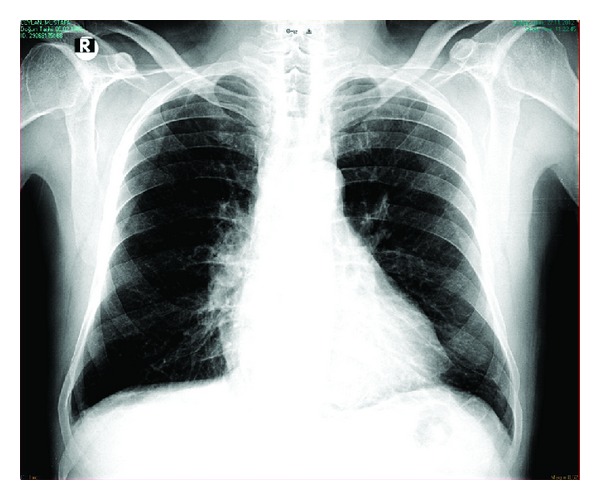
Chest X-ray after three weeks. Complete resolution of pericardial and pleural effusions.
